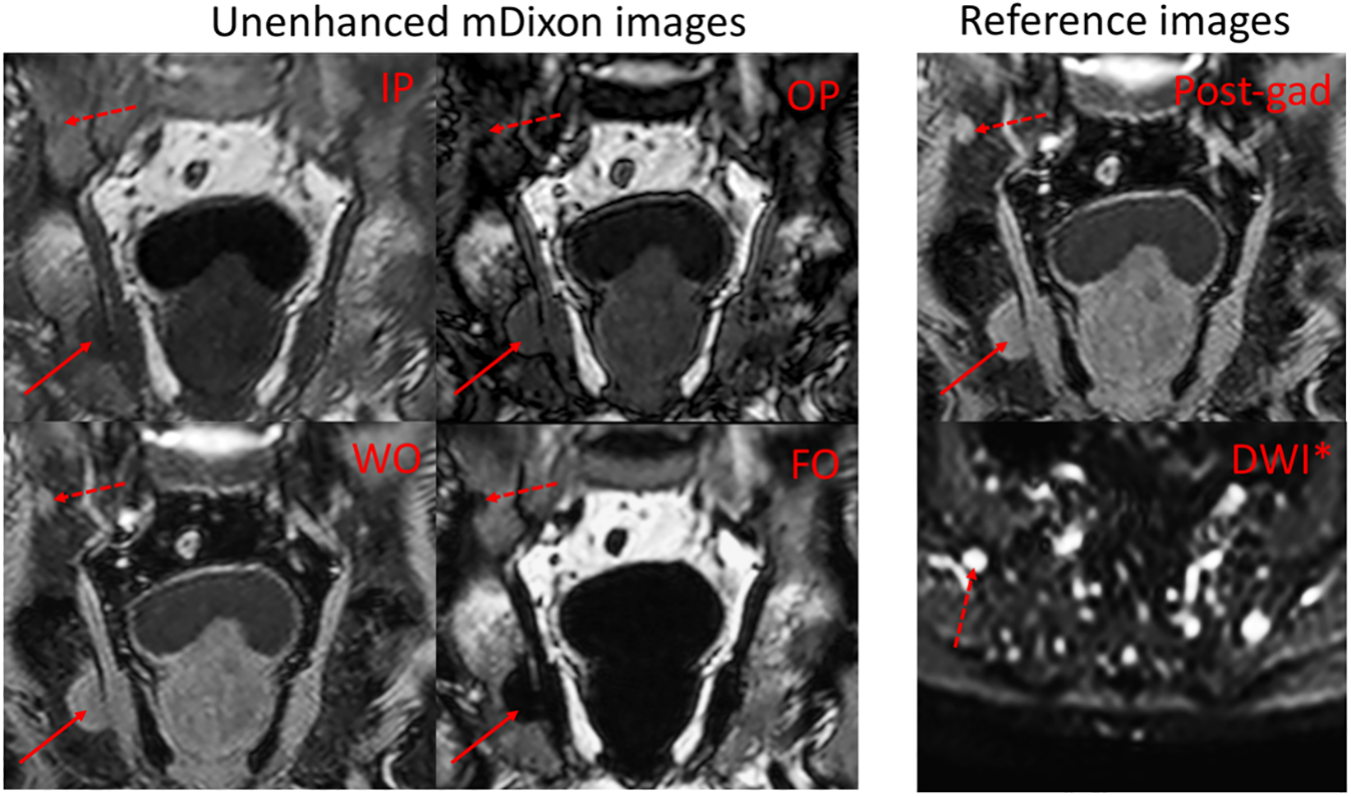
Examples of focal MM lesions. There is a large focal lesion in the right ischium (solid arrow) and a smaller lesion in the right ilium (dashed arrow); both lesions are shown on unenhanced Dixon images (IP, OP, WO and FO) and on the reference images (consisting of DWI and post-contrast). The smaller lesion is less conspicuous on the IP and OP images than on the FO and WO images. *DWI was acquired in the axial plane; the smaller of the two lesions (in the right ilium) is again marked with a dashed arrow.
